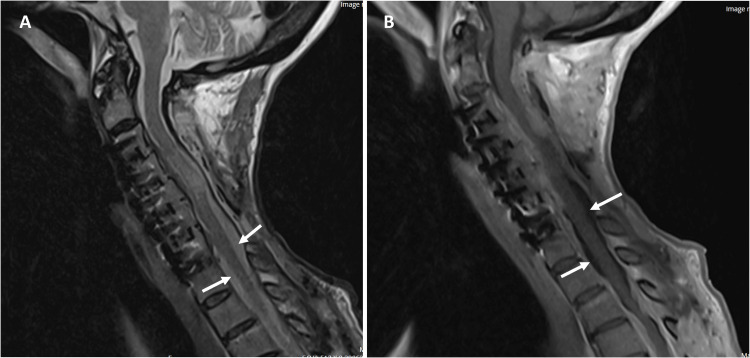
One-month post-operative MRI Follow-up MRI of the cervical spine at one month post-operatively, demonstrating improved mass effect on the spinal cord with improved CSF signal (denoted by arrows) surrounding it, along with diminished contrast-enhancing mass lesion. A T2-weighted sagittal image is depicted (A) as well as a post-contrast T1-weighted image (B).
